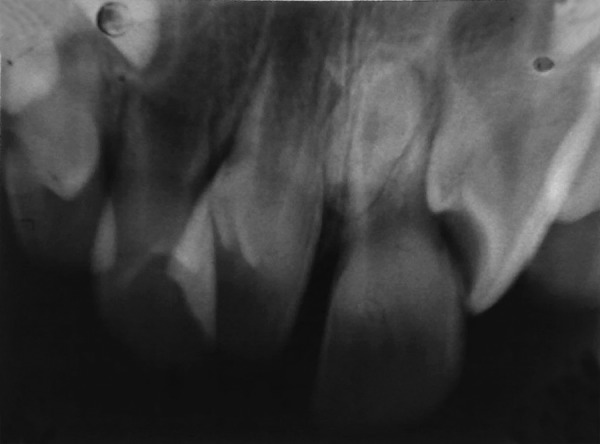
Intraoral periapical radiograph revealing impacted supernumerary tooth (case IV)
